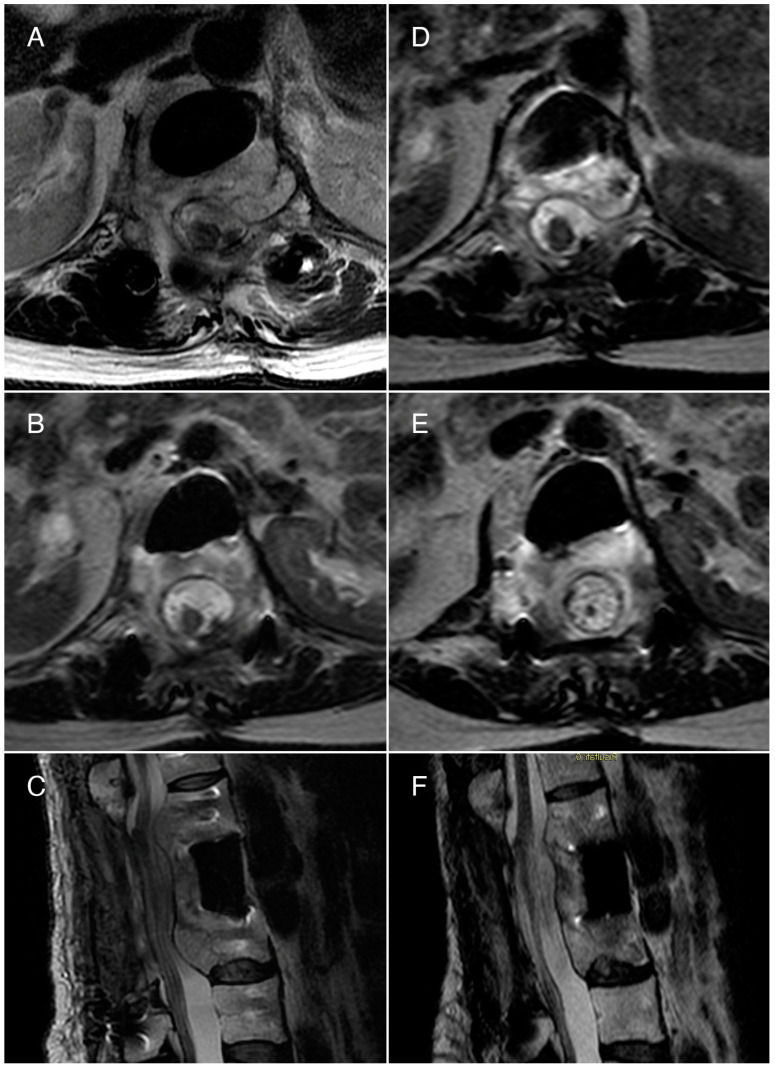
Case 1: T2-weighted MRI scans. (A,B) Axial views and (C) sagittal view performed before the first ECT treatment. The axial view at the L2 level shows a hemangioma causing compression of the dura and the cauda. The sagittal view reveals the hemangioma’s extensive invasion into the epidural space, both above and below the surgically removed L1 vertebral body, at the levels of T12 and L2. (D,E) Axial views and (F) sagittal view performed 12 months after the first ECT show a reduction of the compression caused by the hemangioma.
